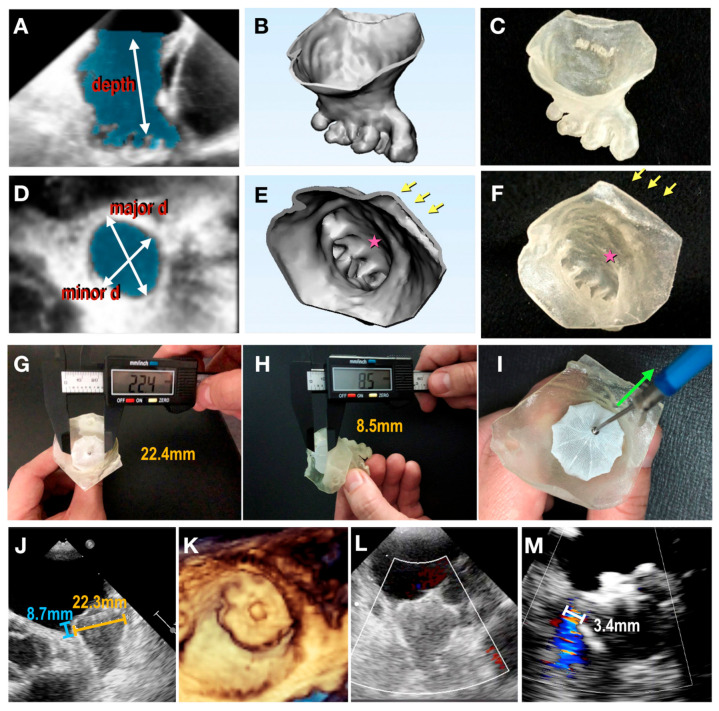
Three-dimensionally printed patient-specific models based on echocardiographic images. (A–F) From 3D transesophageal echocardiography (TEE) image to 3D physical model. (A,D) Segmentation of left atrial appendage (LAA) (shaded area) based on 3D TEE data. Measurements regarding the major and minor ostial diameters and depth of the LAA were taken. (B,E) Creation of a digital object. (C,F) Three-dimensional printed physical model made of tissue-mimicking material. Arrows denote pulmonary vein ridge; stars denote appendicular trabeculations. (G–I) Modifying the size of the 3D model. (G) Device compression and (H) protrusion in 3D model measured using a digital caliper. (I) Tug test for stability. (J) Device compression and protrusion measured in a clinical procedure. (K) Three-dimensional TEE en face view of final device position. (L) Color Doppler assessment showing no peridevice leaks. (M) In another case, color Doppler assessment revealed a residual leak with a jet width of 3.4 mm. Reprinted with permission under open access from Fan et al. [112].
